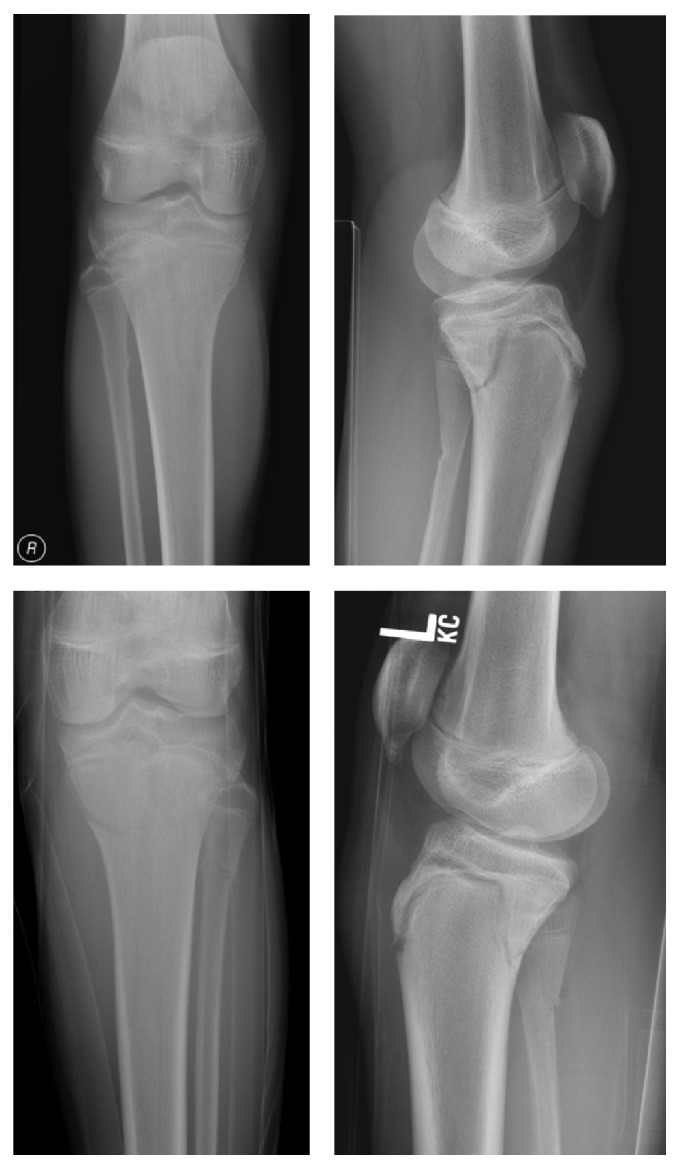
AP and lateral radiographs of both knees.
